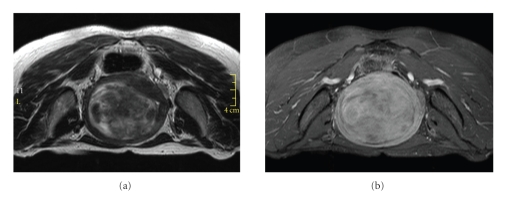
Patient screening MR images. (a) Axial T2-weighted image showing a heterogeneous fibroid, hyper intense relative to the uterine wall. (b) Contrast enhanced Axial T1-weighted screening MR image, showing enhancement of the fibroid.
